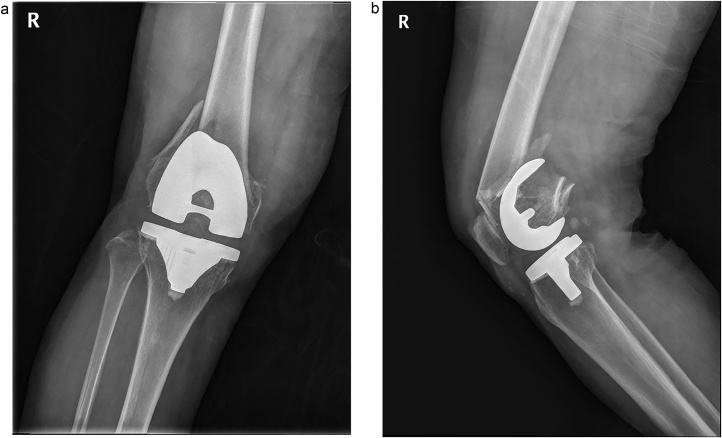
Preoperative radiographs showed that fracture line extends more distal than proximal border of an implant. (A) anterioposterior radiograph (B) lateral radiograph.
